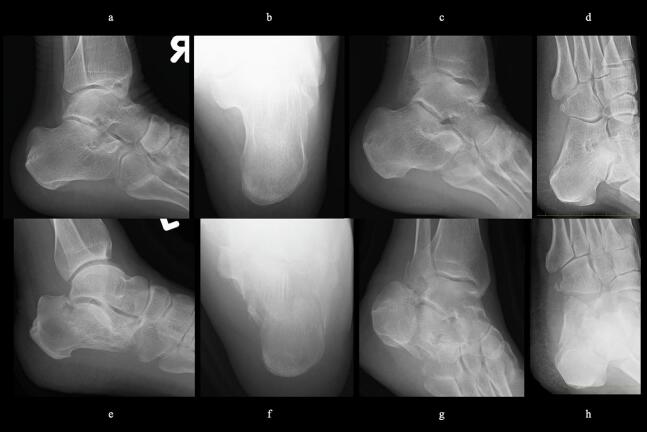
Simple X-ray at the time of injury. a:Lateral view of the affected calcaneus. b:Axial view of the affected calcaneus. c:Broden view on the affected side. d:Oblique view of the affected foot. e:Lateral view of the normal side. f:Axial view of the normal side. g:Broden view of the normal side. h:Oblique view of the normal side.
